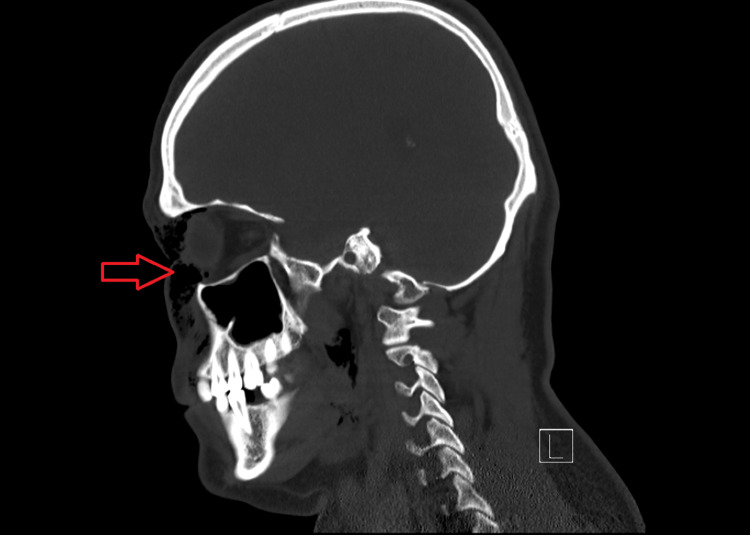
Sagittal CT view showing orbital subcutaneous emphysema
